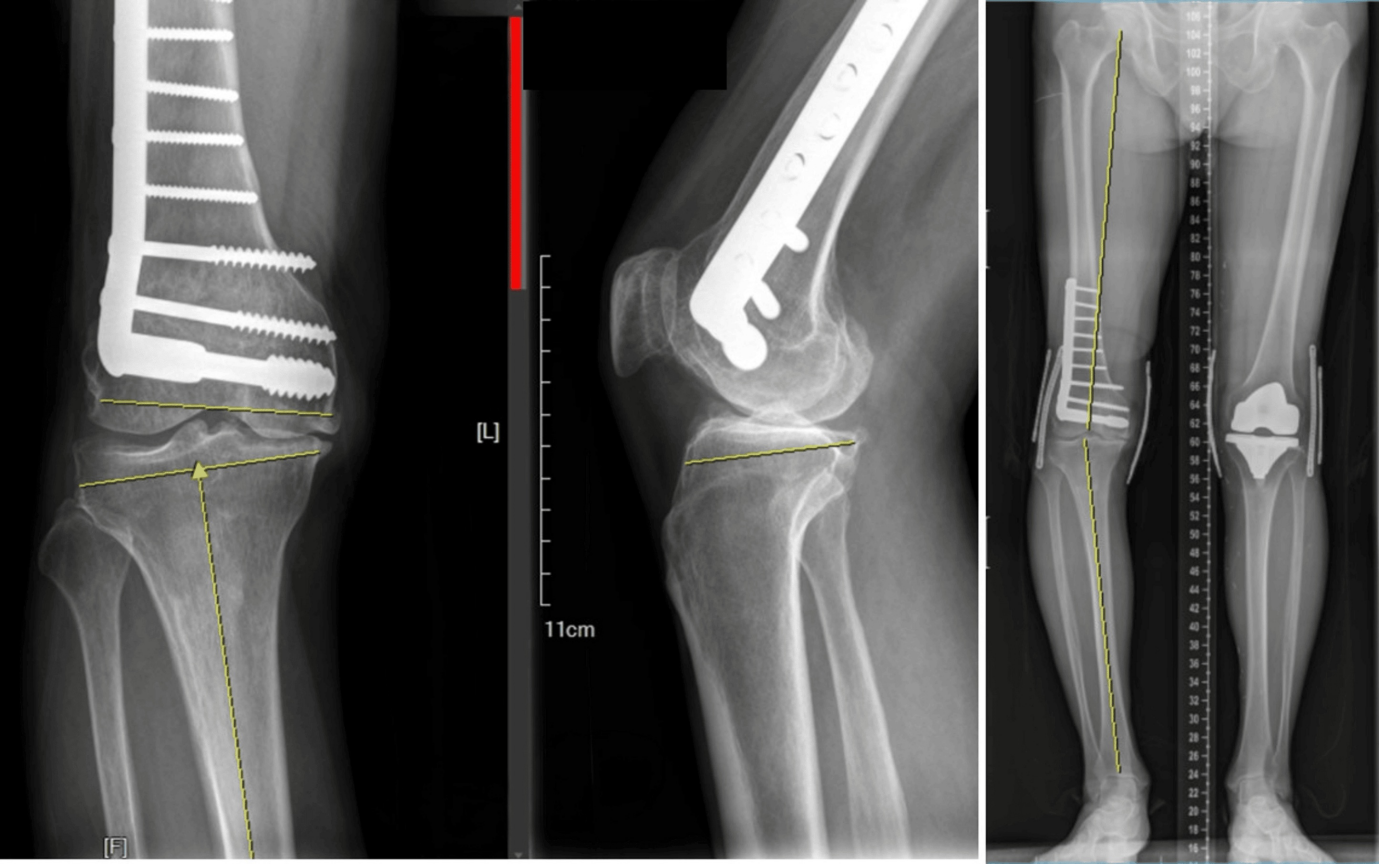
Preoperative radiographic planning Critical bone cuts on the proximal tibia and distal femur are planned using images, including standing AP, lateral projections, and long-leg films (scanogram). The necessity of removing prior hardware is also evaluated. For planning the proximal tibial cut, a horizontal line perpendicular to the tibial shaft is drawn according to the depth set within 2 mm of the most damaged part on the tibial plateau (AP view), as is our practice in conventional primary TKA. The determined shape helps the surgeon control the orientation of their oscillating saw, usually thin in the medial part and thick in the lateral part in cases of genu varum. Additionally, the lateral projection helps the surgeon assess the posterior tibia slope and adjust the inclination of the saw accordingly during the osteotomy. Typically, the osteotomized fragment is thick anteriorly and thin at the back when there is a positive tibial slope. Extreme left image: anterior-posterior (AP) view; image in the center: lateral view; extreme right image: scanogram TKA: total knee arthroplasty
